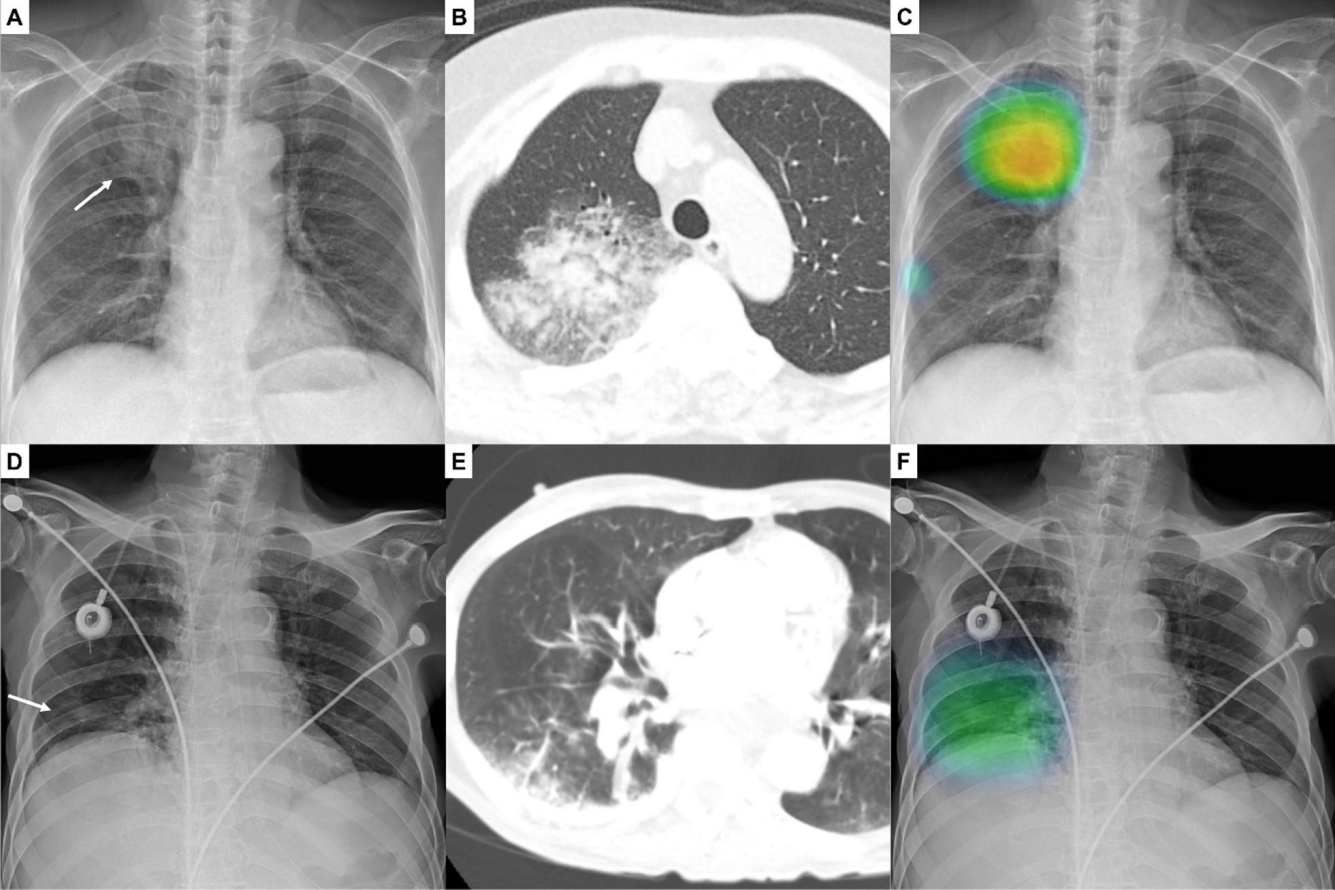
Identification of pneumonia on chest X-ray (CXR) using the computer-aided detection (CAD) system
